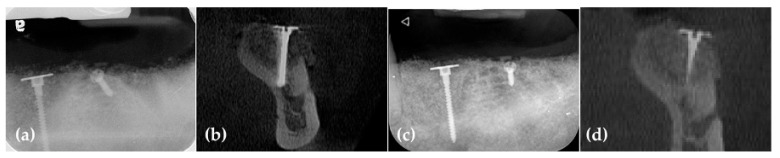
(a) A postoperative radiograph shows the grafted bone material placed over the WHTPS; (b) A cross-sectional CBCT image taken immediately after surgery reveals the grafted bone material surrounding the bone builder; (c) At 5 months postoperative, a radiograph shows that the grafted bone material remains well-maintained without collapse, extending up to the cover screw of the WHTPS; (d) Cross-sectional CBCT images at 5 months of healing demonstrate that the grafted bone material surrounding the bone builder remains intact. The inverted letter a and the triangle indicate orientation marks of the radiograph.
